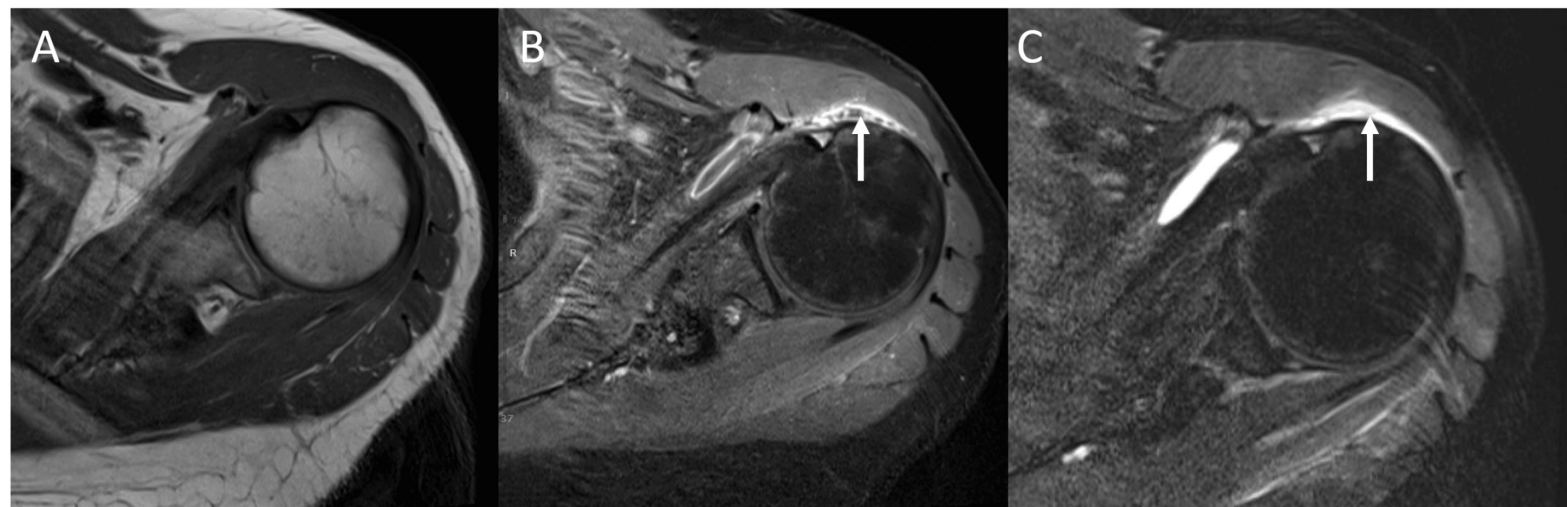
Left shoulder MRI with and without contrast demonstrated subacromial bursitis (A) Axial T1 phase pre-contrast; (B) Axial T1 phase post-contrast demonstrated septation; (C) Axial T2 phase demonstrated synovial thickening and oedema within the subacromial bursa; arrows point to respective features (B-C).
